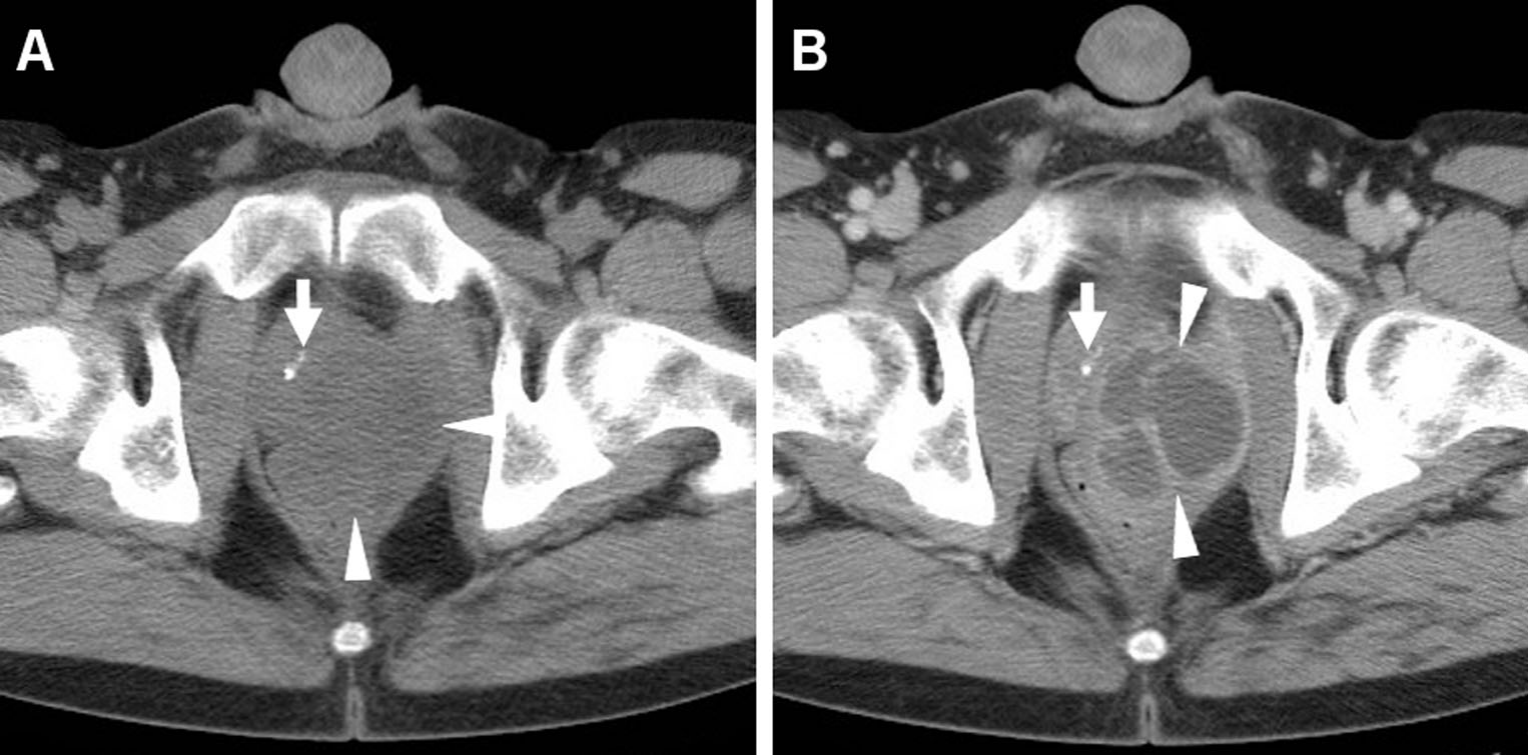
Prostatic abscess in a 48-year-old man with perineal pain and abnormally increased CRP. Axial unenhanced (a) and post-contrast (b) CT images showed mild asymmetric prostatic enlargement, occupied by a 4-cm septated fluid-like collection (*arrowheads*) with peripheral and septal enhancement. Note displacement of periurethral calcifications (*thick arrows*) from midline. Ultrasound-guided transperineal drainage confirmed *Escherichia coli* infection
